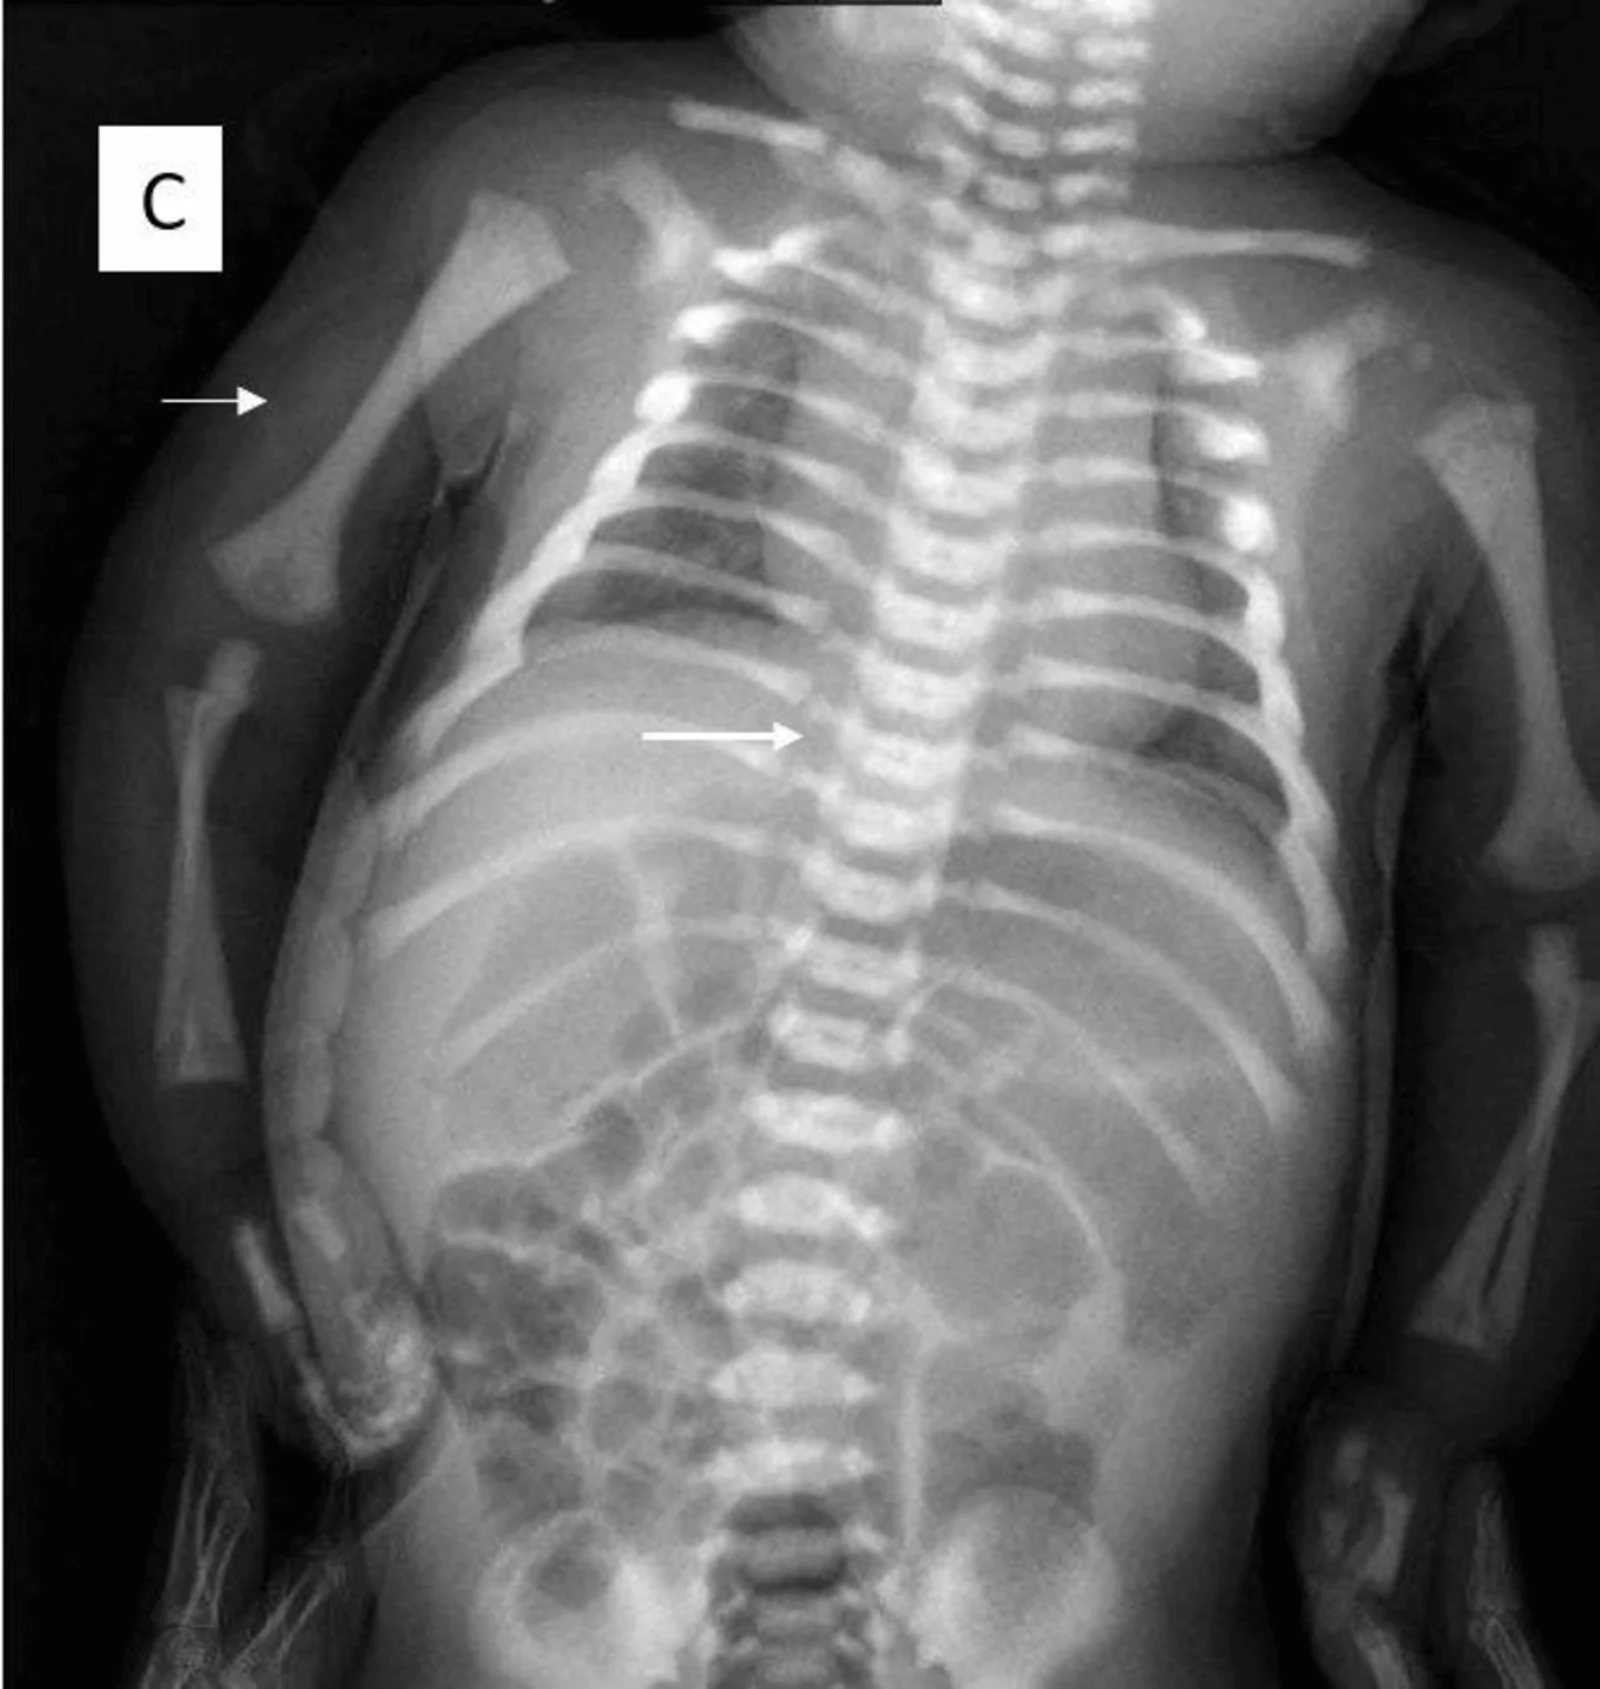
Chest radiograph demonstrating diffuse skeletal sclerosis with obliteration of the normal corticomedullary differentiation. A healing fracture of the right humerus is noted (upper arrow), and generalized increased vertebral density is evident (lower arrow), reflecting the diffuse skeletal involvement of infantile osteopetrosis. The patient had both a right clavicular fracture at birth and a right humeral fracture identified on follow-up imaging
